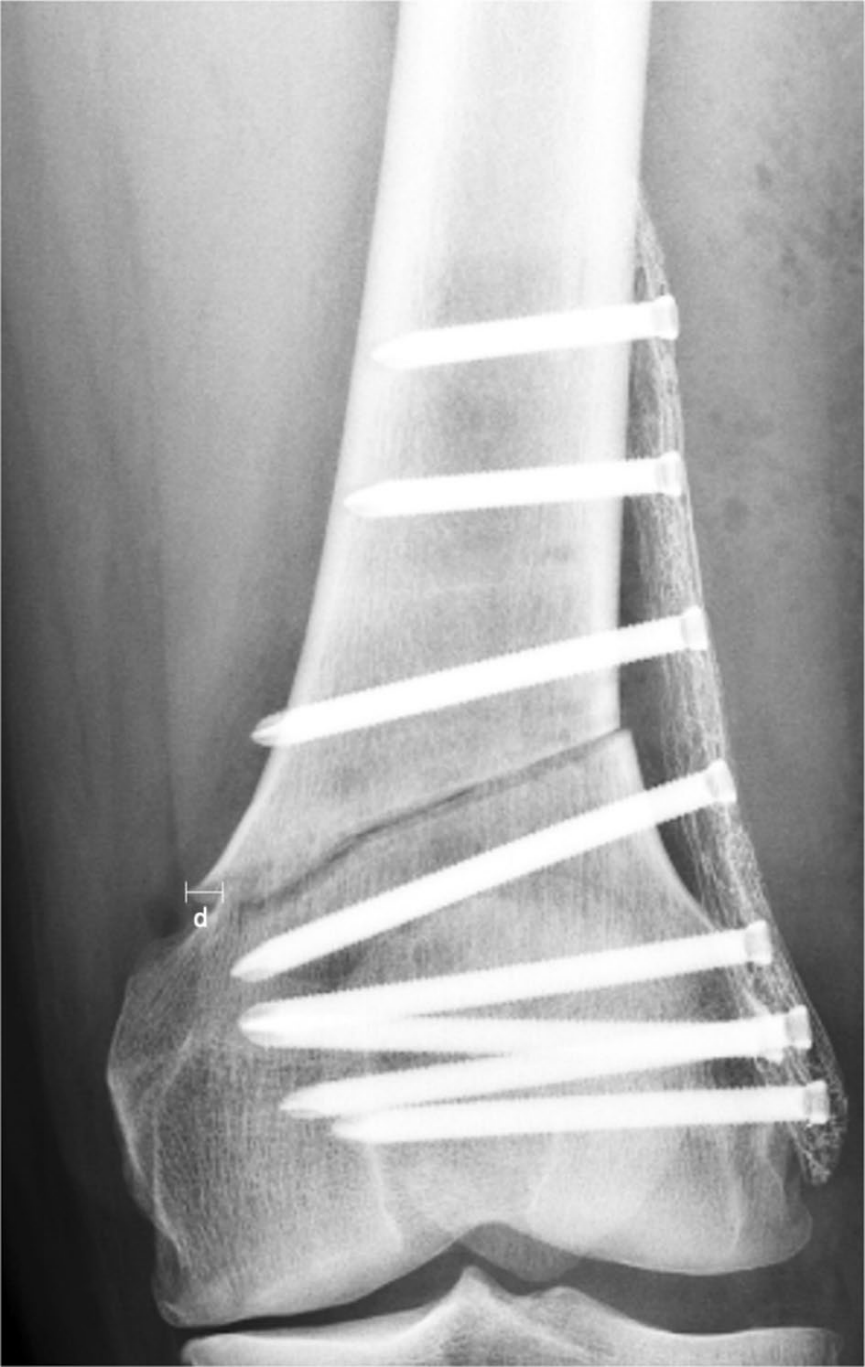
Mediolateral dislocation after medial cortical hinge fracture. A standard AP radiograph taken on the first postoperative day after biplane LCW-DFO using a TomoFix™ (DePuy Synthes, Raynham, MA, USA) locking compression plate is showing a mediolateral dislocation of > 2 mm in the coronal plane caused by a medial cortical hinge fracture. Distance d; horizontal distance between the proximal and distal medial cortex
